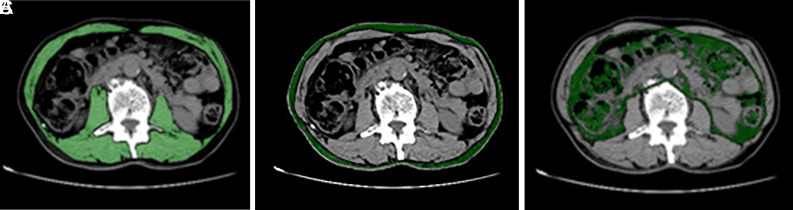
Skeletal muscle area measurement range (−30 to 150 HU). (A), SFA measurement range (−190 to −30 HU) (B), and VFA measurement range (−190 to −30 HU), (C) show body composition measurement on abdominal CT (at the level of the third lumbar vertebra). SFA, subcutaneous fat area; VFA, visceral fat area; CT, computed tomography; HU, Hounsfield unit.
